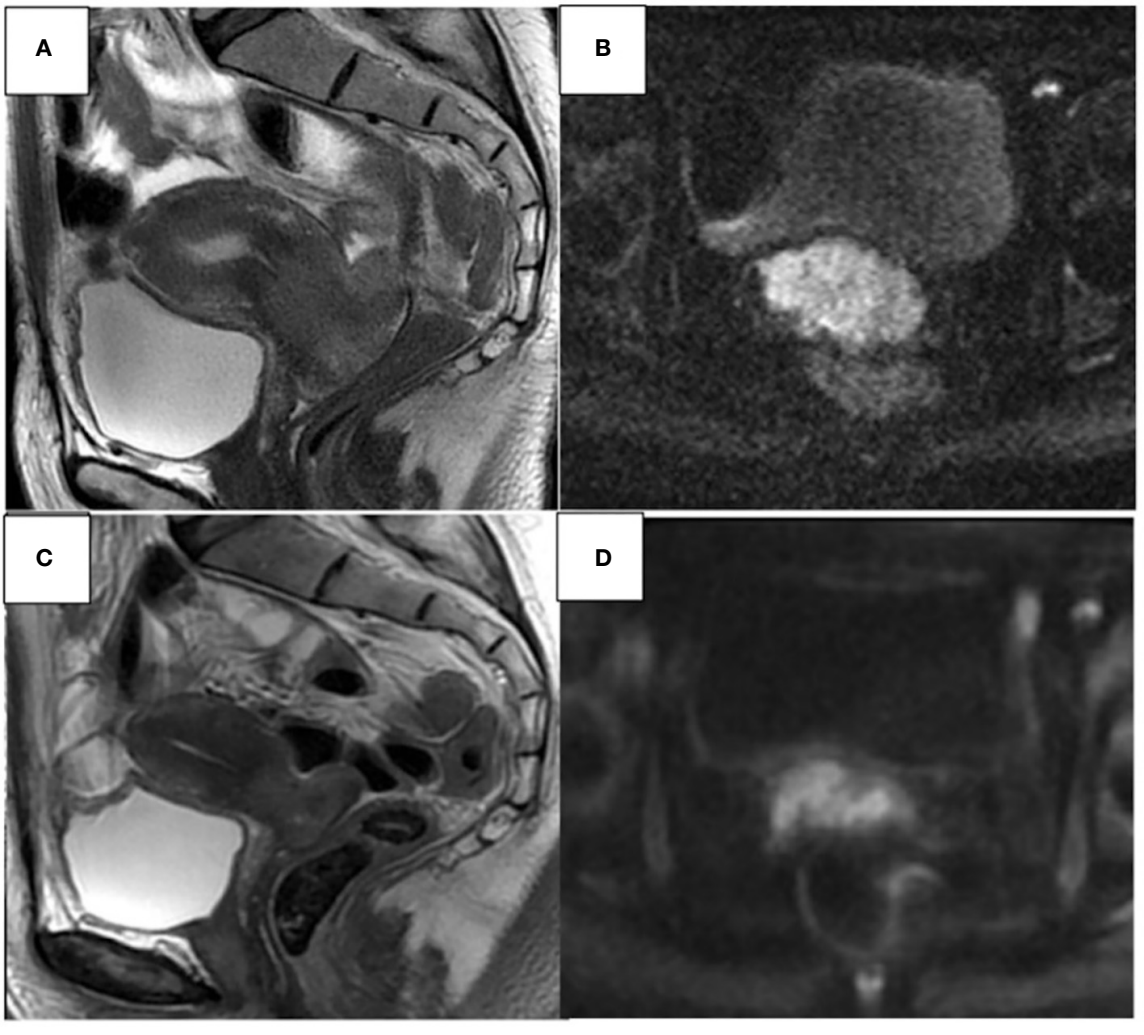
48- year-old woman. Sagittal T2W and axial DWI MR images show an invasive CC extending from the uterine isthmus to the external uterine orifice, laterally infiltrating both vaginal fornices, and extending beyond the stromal ring with extensive infiltration of the parameters. (A, B). Partial response (C, D) approximately 30% reduction of cervical heteroplastic tissue.
